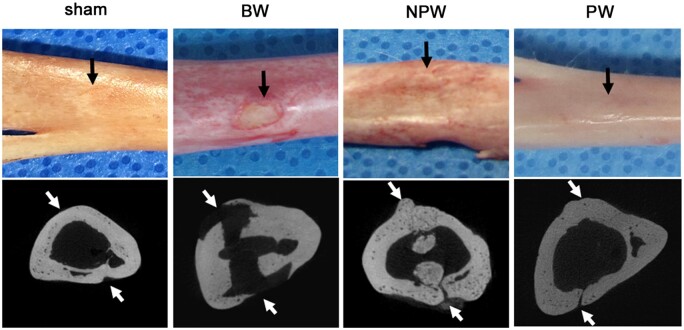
Representative images of tibiae at 16 weeks after surgery. The upper panel shows morphological images, and the lower panel shows micro-computed tomography images. Black and white arrows show the positions of bone defects.
